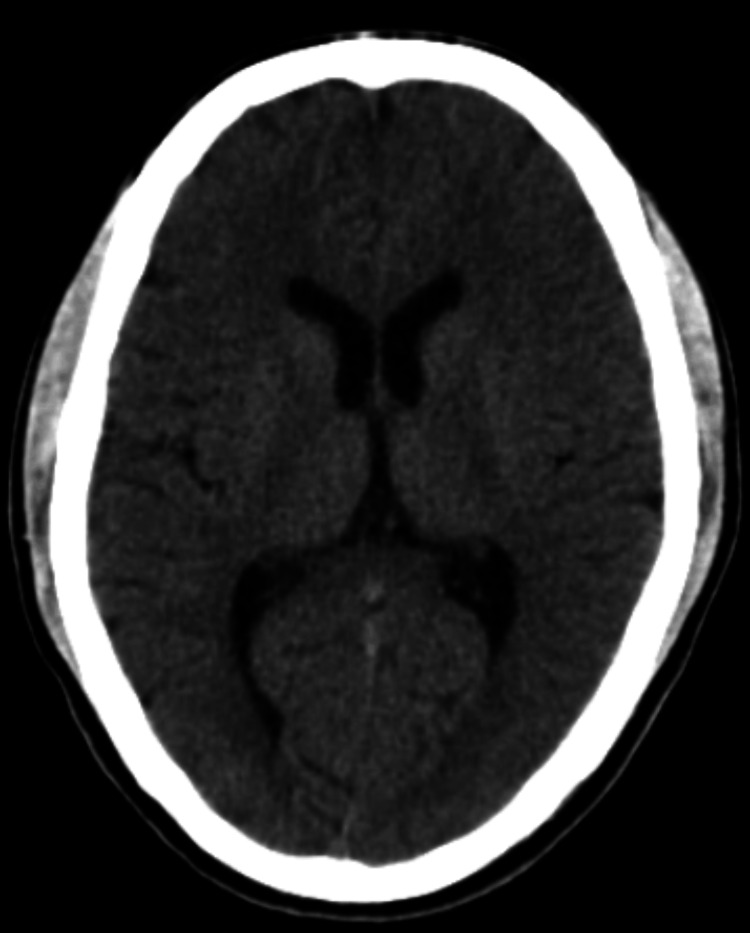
Follow-up CT brain (axial view) done one month after discharge CT: computed tomography
